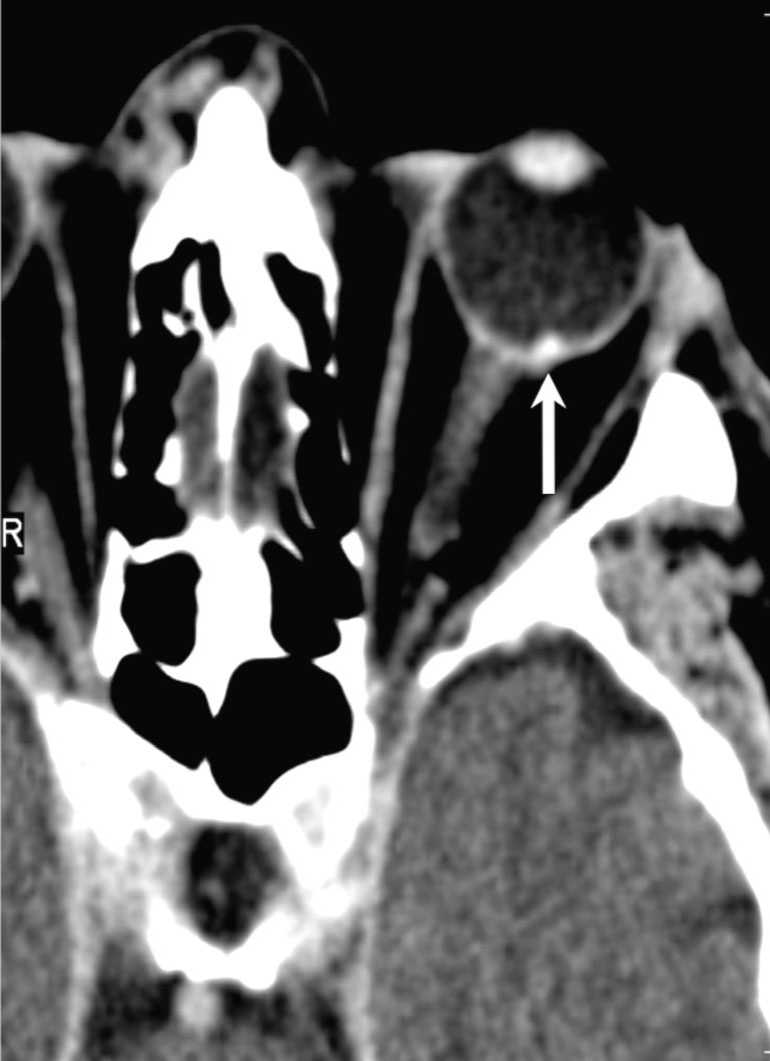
50-year-old male patient diagnosed with a left-sided uveal melanoma on ophthalmoscopy. a. Axial contrast-enhanced CT image shows a tiny, avidly enhancing nodule (arrow) along the choroid
